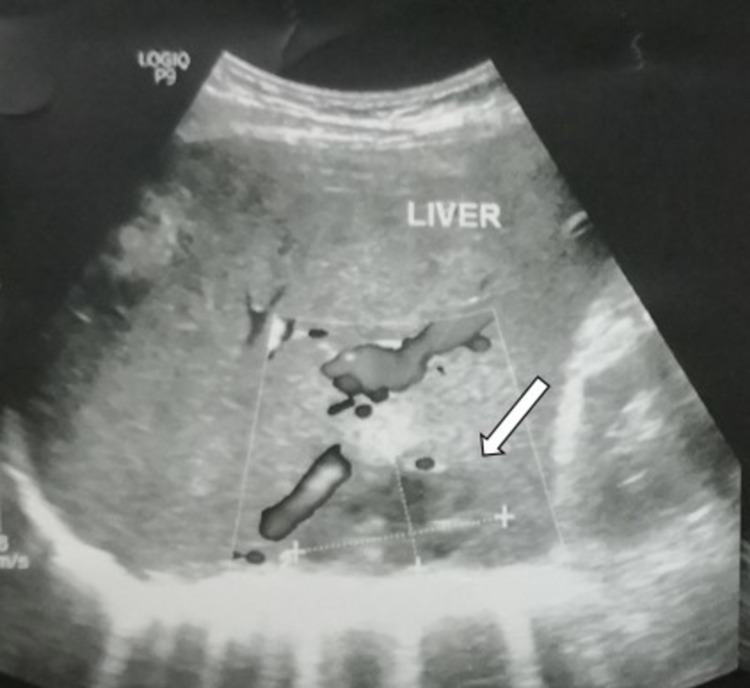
Ultrasound hepatobiliary system showing a hypoechoic lesion (arrow) in liver segment 6.
